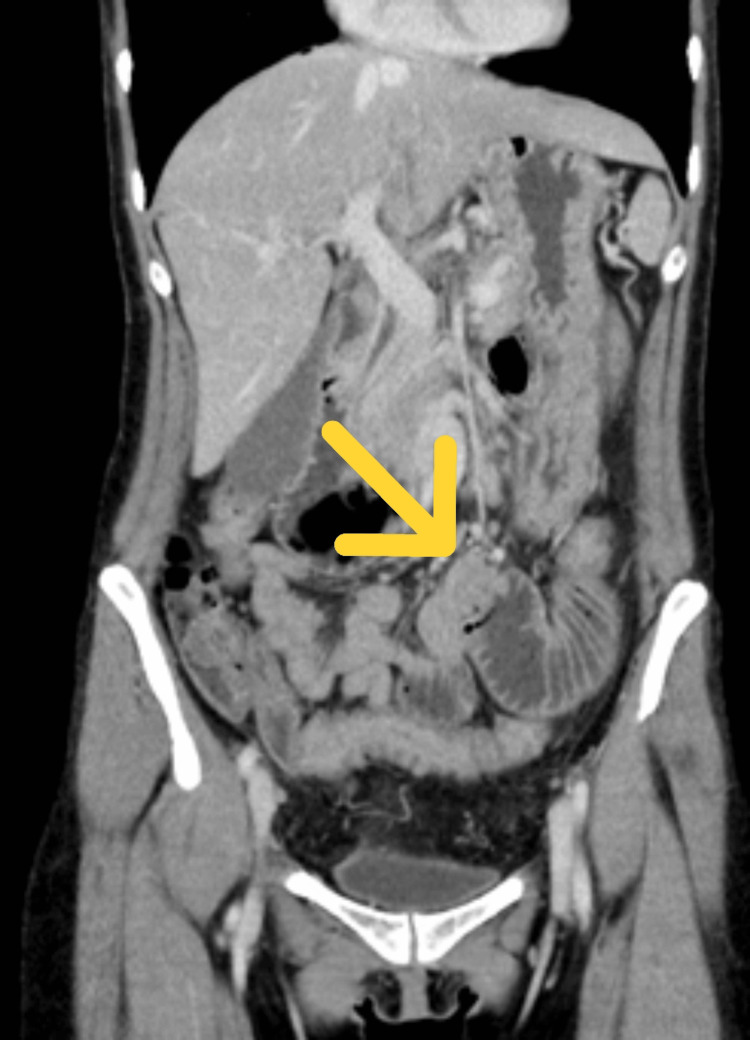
Wall thickening of the upper jejunum Coronal CT reconstruction demonstrating the extent of upper jejunal wall thickening and proximal bowel dilation. The arrow indicates wall thickening of the upper jejunal wall.
